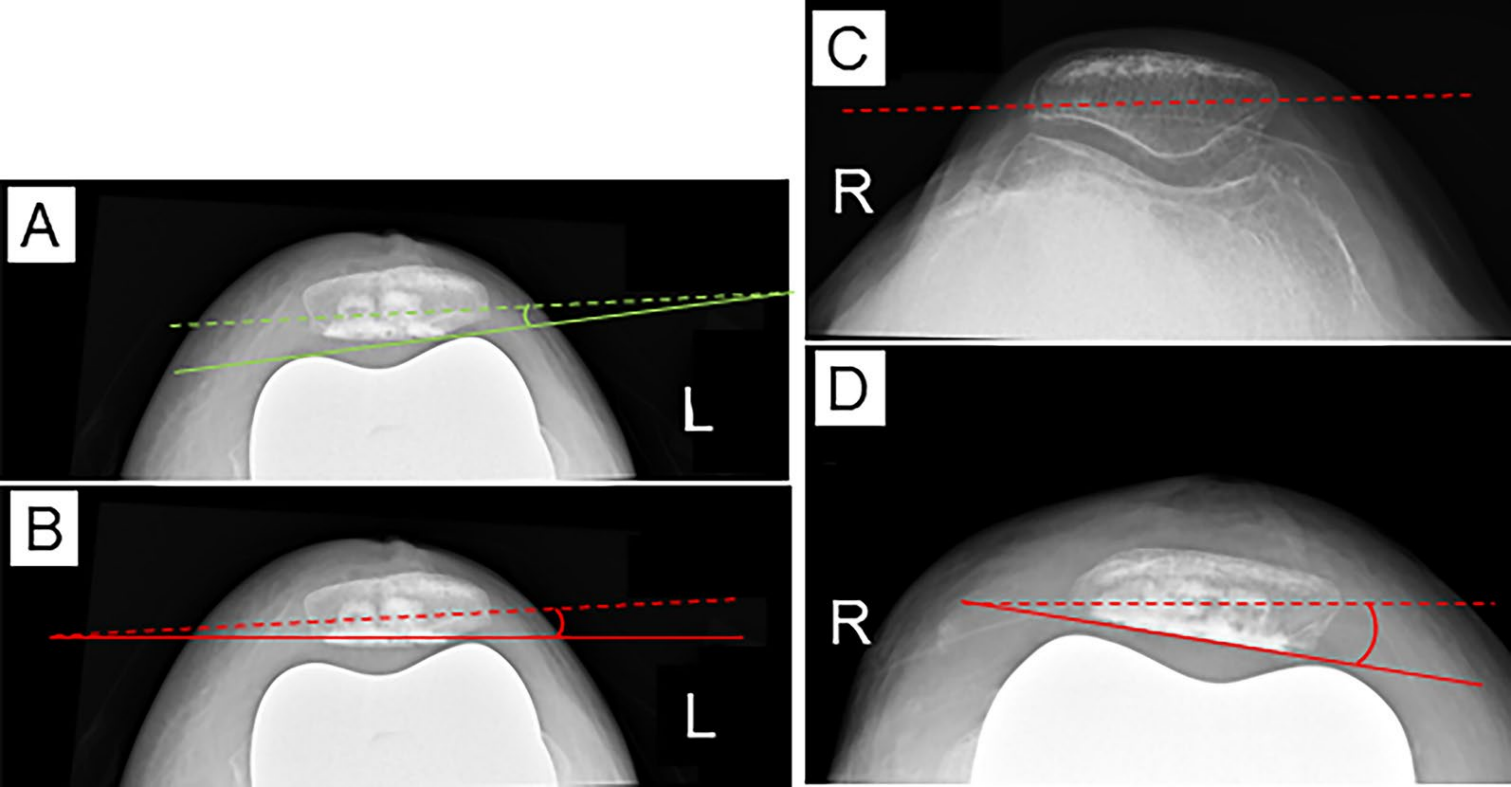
Radiographic measurement using the skyline view. A The PT is defined as the angle of a line drawn along the long axis of the patella (green dashed line) with respect to a line connecting the anterior aspect of the femoral condyles or femoral component (green solid line), with an external rotational angle being considered as positive. Postoperative PT of the left knee is shown in A. B–D The PRA was defined as the angle of a line drawn through the patellar prosthesis–bone interface (red solid line) with respect to a line along the long axis of the patella (red dashed line), with an external rotational angle being considered as positive. The PRA of the left knee was 2.3° (B). Based on the pre- and postoperative radiographs of the right knee, the PRA was calculated as −8.9° (C, D). *PRA* patellar resection angle, *PT* patellar tilt
